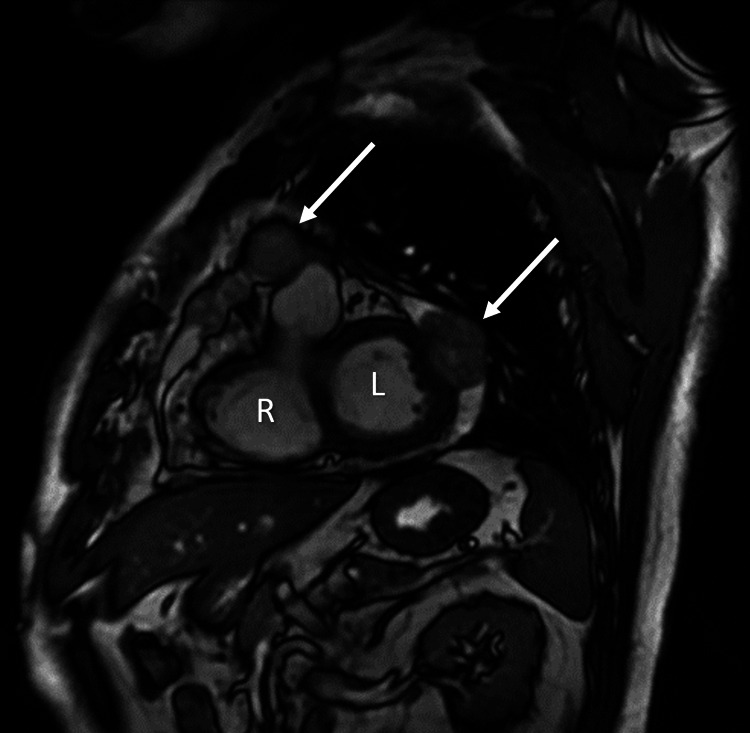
Cardiac magnetic resonance image (MRI) identifying mass-like right atrial and pericardial lesions (arrows), right ventricle (R), and left ventricle (L)
